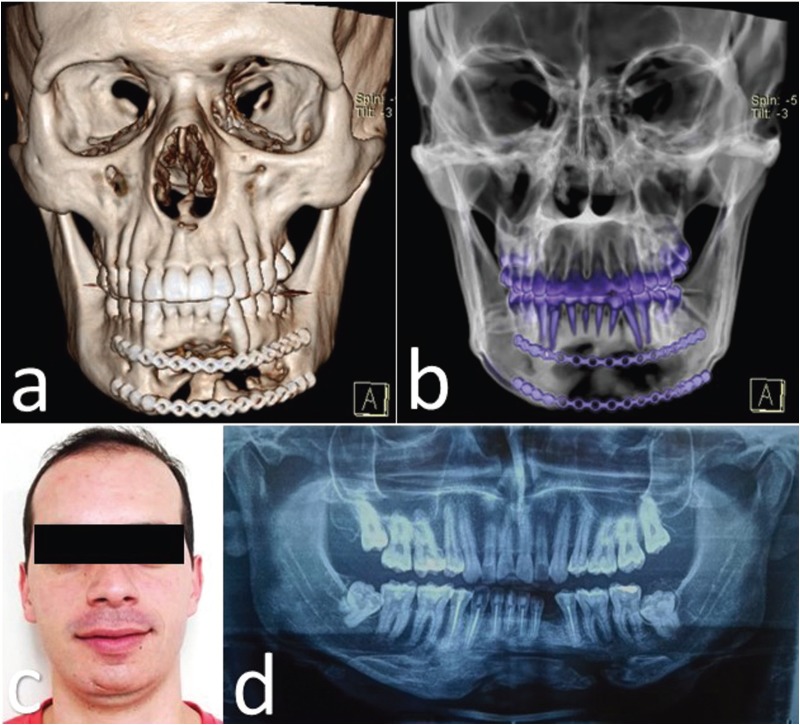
a,b. 3D reconstruction of front-view computed tomography 6 months after the postoperative check-up showing adequately positioned titanium plates and ongoing bone remodeling. c. Twenty-four months of postoperative follow-up, with no facial asymmetry. d. Panoramic radiograph after 24 months of postoperative follow-up showing bone defect in the surgical site associated with corticalization of the edges and absence of residual lesions.
